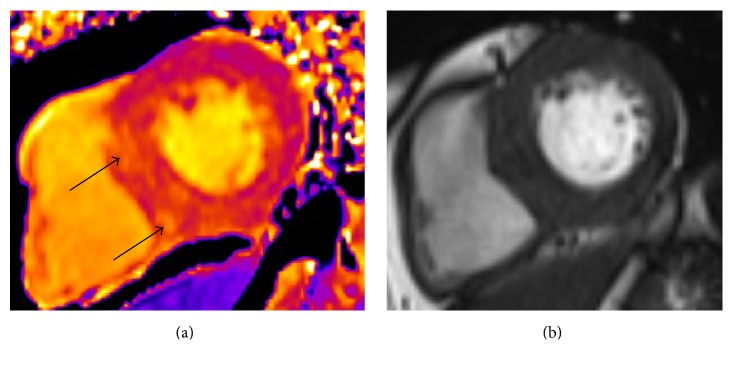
(a) Short-axis midventricular native T1 map of a dialysis patient. Black arrows show areas of discretely increased signal intensity likely to represent myocardial fibrosis. (b) Corresponding short-axis midventricular plain cardiac MRI cine image of the left ventricle of the same dialysis patient. No tissue abnormality visible on plain MR imaging.
